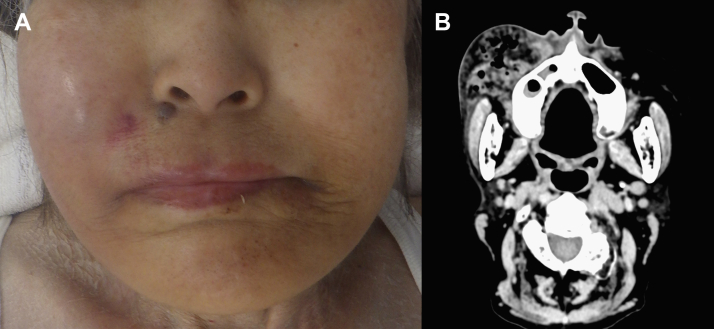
Right buccal subcutaneous abscess. (A) Clinical photograph demonstrating diffuse swelling of the right cheek with overlying erythema. (B) Axial computed tomography demonstrating a subcutaneous abscess with gas formation in the right buccal region.
